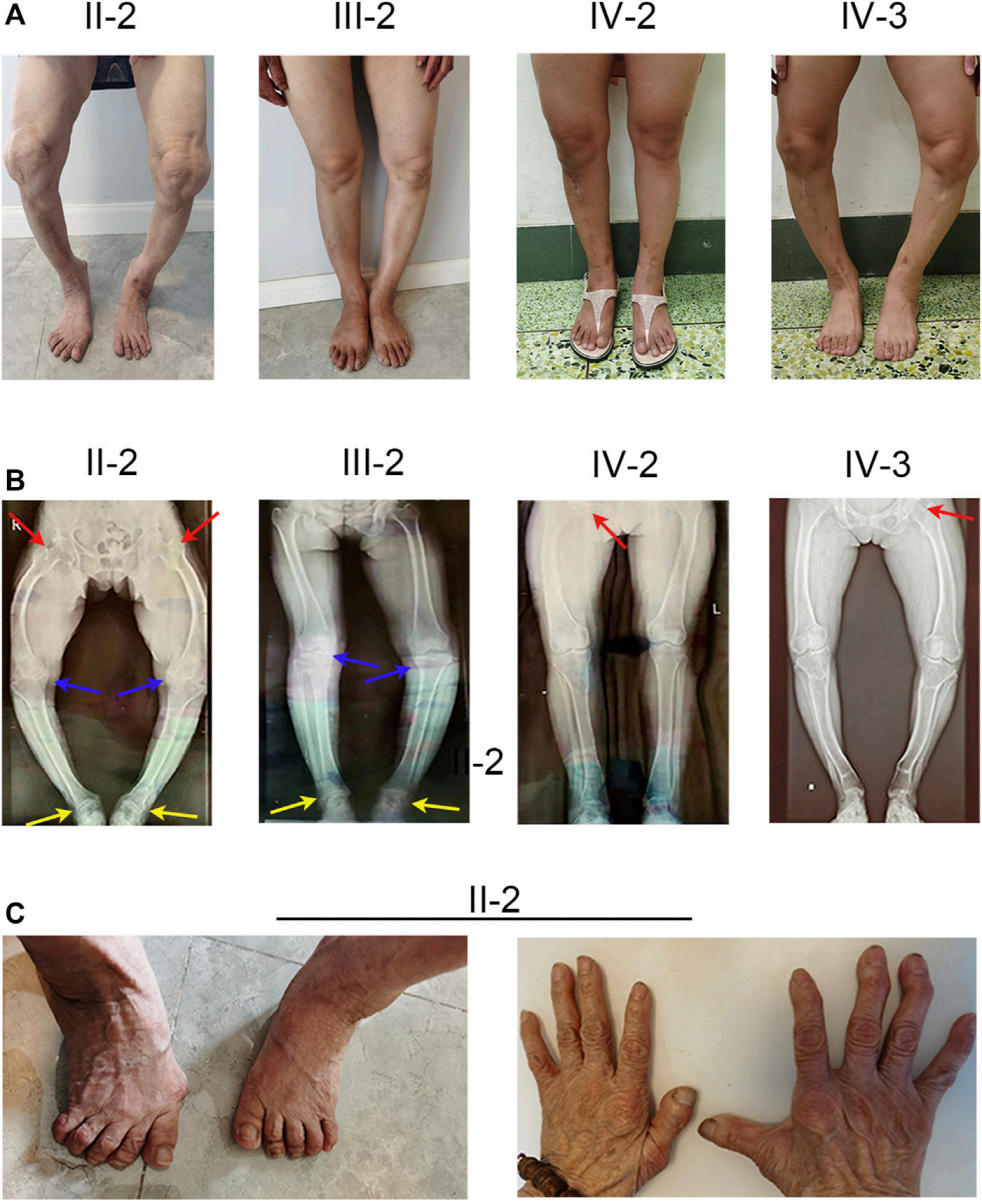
Phenotype and X-ray autoradiographs of the family. (A) Clinical phenotype of the affected individuals in the family. The patients present with short stature and obviously bowed legs. (B) The radiographs of the lower limbs of the patients show varying degrees of genu varum and osteoarthritis, with osteophytes on the joint margins (indicated by arrows. hips, red; keens, blue; ankles, yellow). (C) Patient II-2 exhibits severe joint deformity of both hands and feet due to the lack of earlier treatment.
